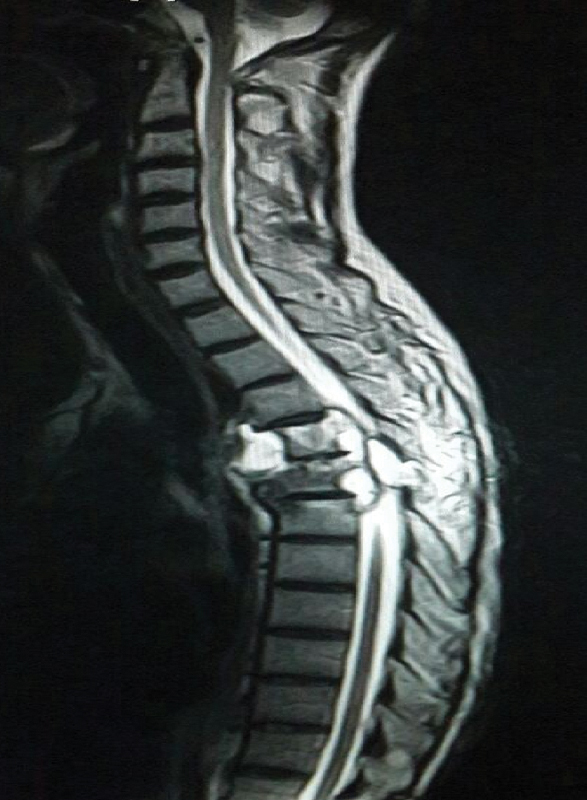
Magnetic resonance imaging showing abnormal signal changes in the T4 and T5 disks and adjacent vertebral bodies with associated prevertebral and epidural soft tissue abnormalities.
